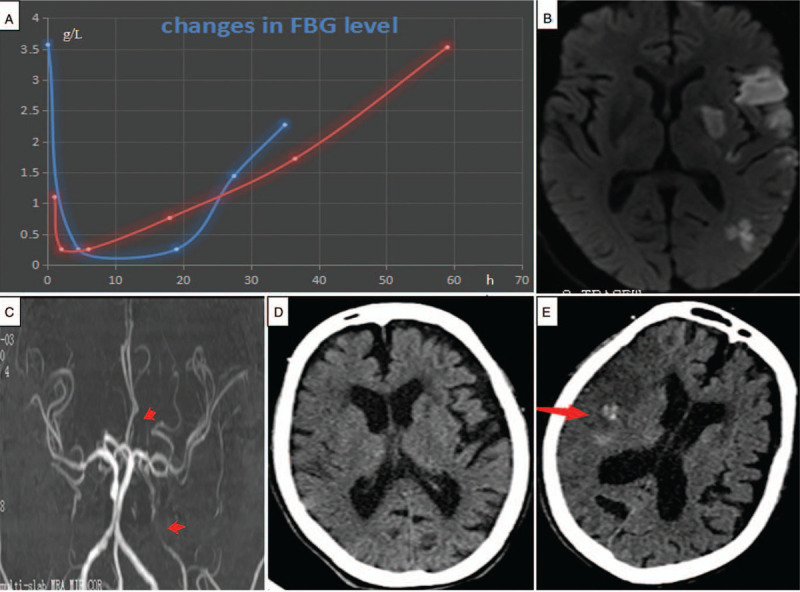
Changes in fibrinogen levels and neuroimaging results. (A) Changes in fibrinogen (FBG) levels in patient 1 (blue line) and patient 2 (red line) after intravenous thrombolysis (IVT) with recombinant tissue plasminogen activator (rt-PA). FBG levels sharply decreased immediately after IVT, and the peak status was sustained for hours, followed by a slight increase. Brain magnetic resonance (MR) diffusion-weighted imaging (B) shows multiple abnormal signals in the blood supply area of the left middle cerebral artery (MCA), suggesting acute watershed infarction in patient 1. Brain magnetic resonance angiography (C) shows severe stenosis of the left internal carotid artery (arrow) and signs of recanalization of the left MCA in patient 1. (D) Brain computed tomography (CT) before IVT shows a few old cerebral infarctions in bilateral subcortex areas in patient 2. (E) Brain CT on the second day after IVT shows a minor hemorrhage (arrow) within the focus of the right frontal and temporal lobe cerebral infarctions in patient 2.
